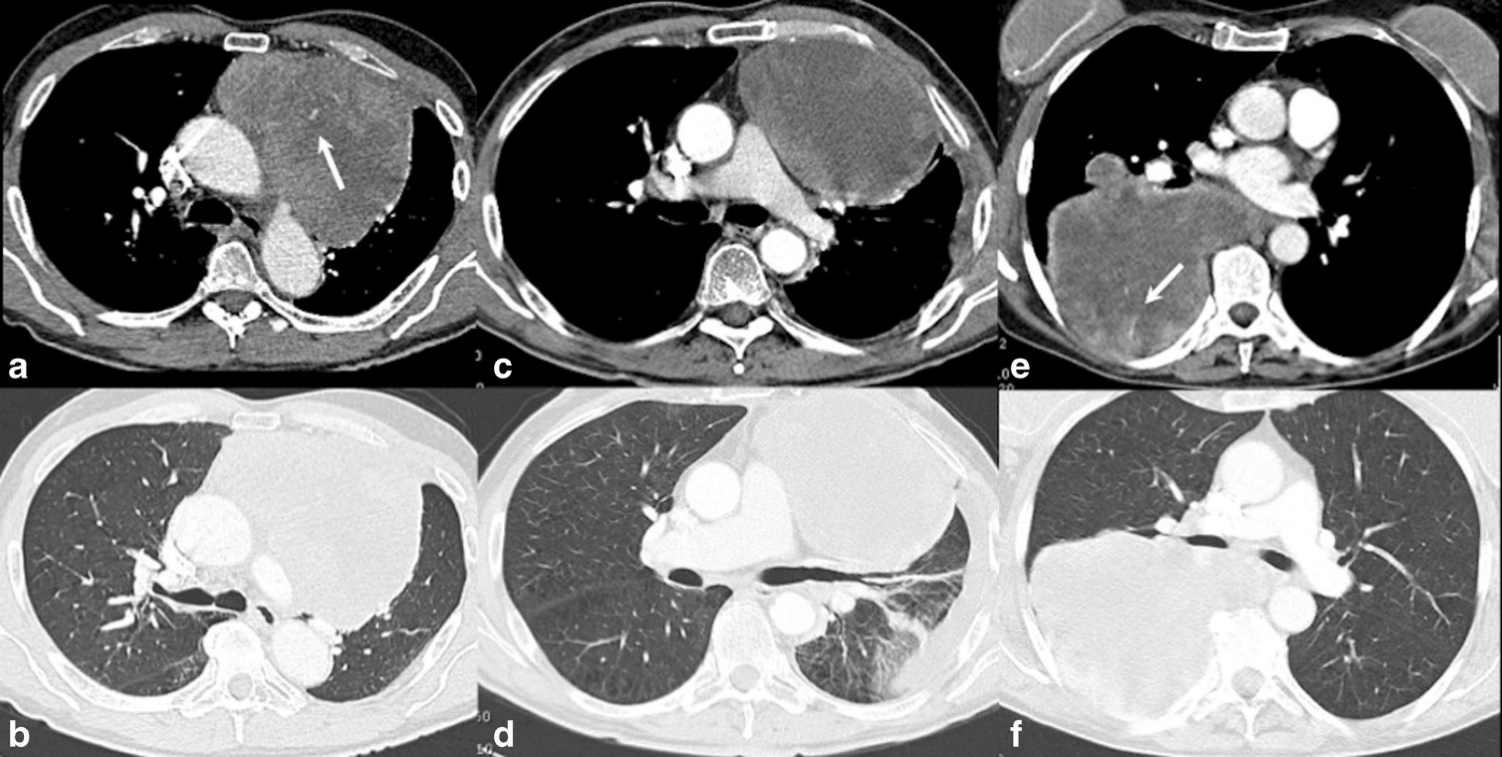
CT images of primary intrathoracic sarcomas. 63-year-old man (a and b) with undifferentiated sarcoma, presenting a large mass (143 mm) in anterior mediastinum, with ovoid shape, smooth margins, heterogeneous enhancement and intratumoural enhancing vessels (*arrow* in a). A 68-year-old man (c and d) with synovial sarcoma of the anterior mediastinum, showing ovoid shape, smooth margins, large necrosis and associated pleural effusion. A 67-year-old woman (e and f) with leiomyosarcoma of posterior mediastinum, mass with irregular shape, lobulated margins, necrosis and also intratumoural vessels (*arrow* in e)
